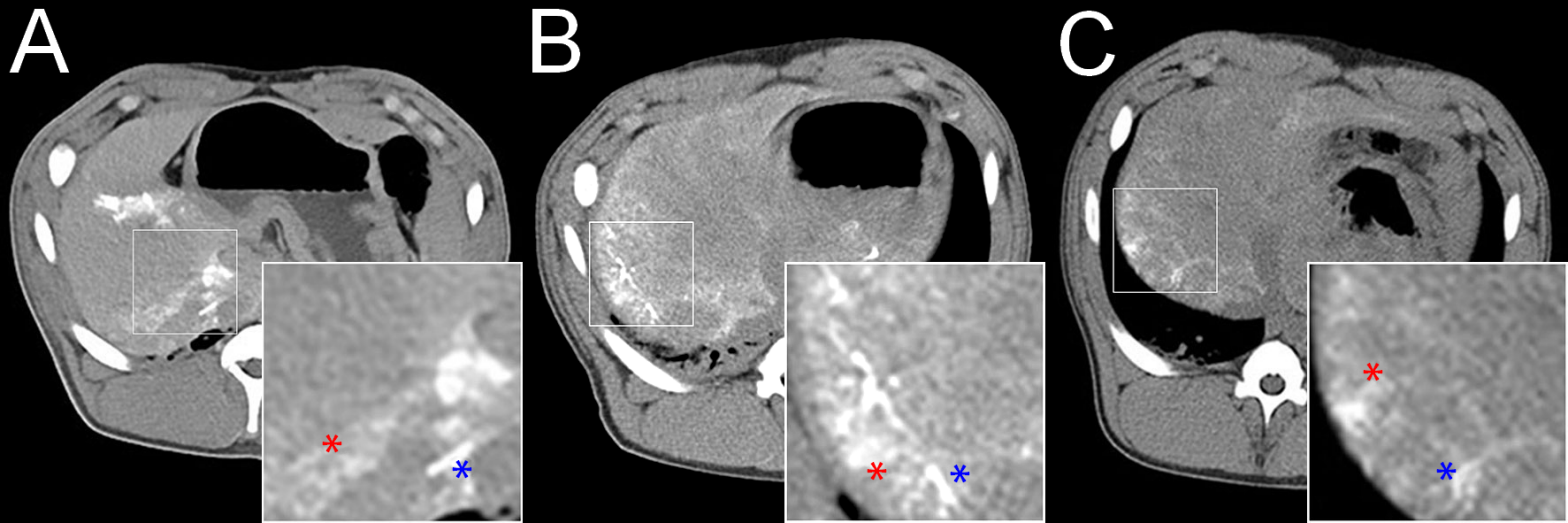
Iodized oil–standard CT findings. A IO.a. Non-enhanced CT 2 hours after embolization with 0.5 mL iodized oil. Linear hyperdensities (blue asterisk) corresponding to an occluded subsegmental artery (arterial enhancement) and patchy hyperdensities (red asterisk) corresponding to occluded interlobular arteries and sinusoids (parenchymal enhancement). B IO.sa. Non-enhanced CT 2 hours after embolization. Note the lower arterial (blue asterisk) and parenchymal (red asterisk) enhancement compared to A. C IO.sa. Non-enhanced CT 7 days after embolization. Note the less intense arterial (blue asterisk) and parenchymal (red asterisk) enhancement compared to B.
